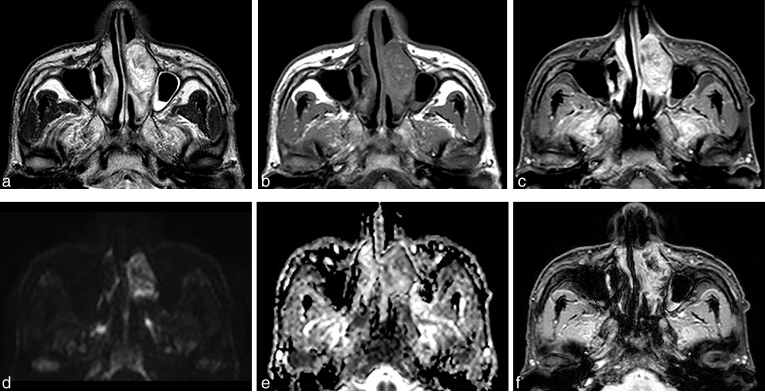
(a–f) MRI scan shows a mass occupying the left nasal cavity. (a) Axial *T*_2_ weighted MRI scan shows heterogeneous high- signal-intensity mass compared with muscle. (b) Axial *T*_1_ weighted MRI scan shows heterogeneous iso-signal-intensity mass and multifocal high-signal foci that suggest a haemorrhagical component. (c). Axial gadolinium-enhanced *T*_1_ weighted MRI scan shows heterogeneous enhancement except for multifocal haemorrhagical foci. (d, e) Axial DWI and ADC MRI scan shows that the mass has no diffusion restriction (average ADC value = 1536.01 mm^2^ s^−1^). (f). Axial gradient-recalled echo MRI scan shows heterogeneous high-signal-intensity mass and multifocal haemorrhagic foci with dark signal intensity (arrow). ADC, apparent diffusion coefficient; DWI, diffusion-weighted imaging.
